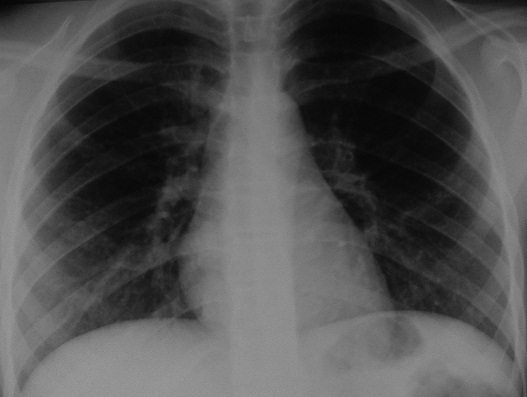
Radiographie thoracique après extraction de l’épingle à foulard
